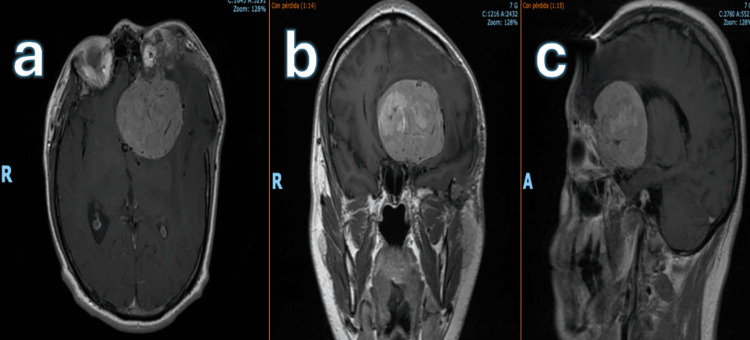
Preoperative MRI, 2024 T1-weighted MRI in axial (a), coronal (b), and sagittal (c) sections showing a heterogeneous lesion with regular margins in the left fronto-orbital region, causing mass effect with midline shift and partial collapse of both the ipsilateral and contralateral frontal horns of the ventricular system.
